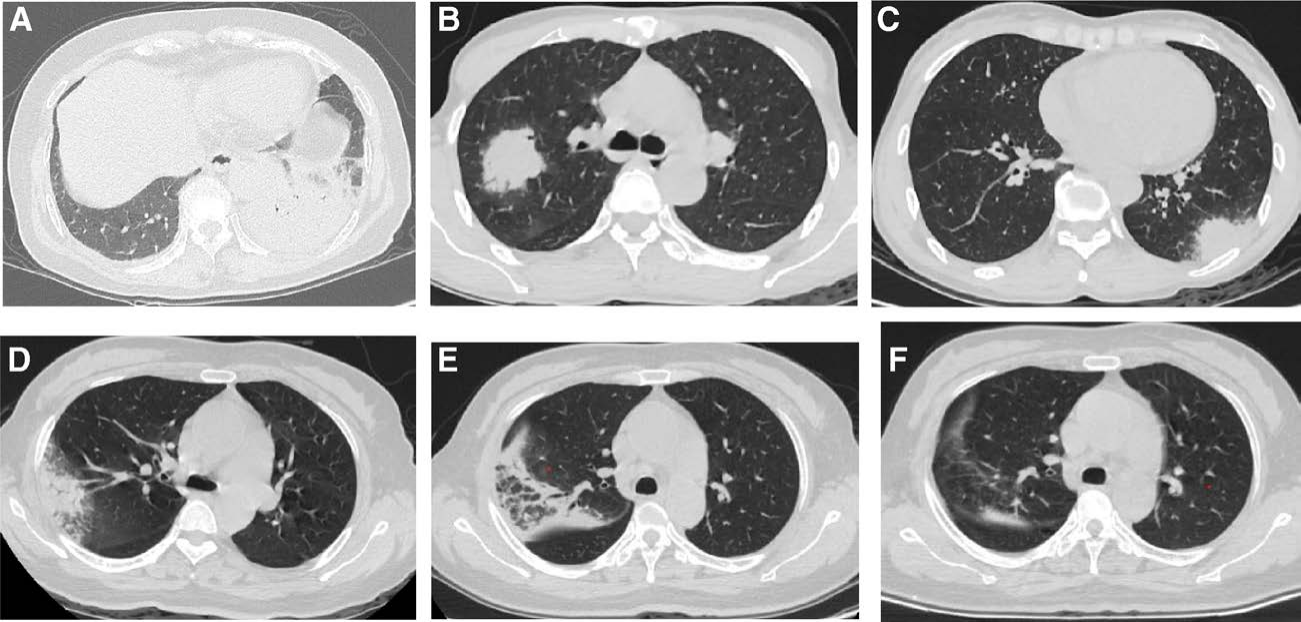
Chest computed tomography (CT) findings of non-severe *Chlamydia psittaci* pneumonia. (A) A 67-year-old female presenting a fever for 3 days, large air-space consolidation in the left lower lobe. (B) A 51-year-old male presenting a fever for 3 days, focal consolidation in the right upper lobe. (C) A 48-year-old female presenting a fever for 2 days, focal consolidation in the left lower lobe subpleural area. (D–F) A 51-year-old female with the initial CT scan performed 3 days after onset showed focal consolidation in the right upper lobe subpleural area (D). The lesions increased, and the scope expanded following treatment with levofloxacin for 7 days (E). Thereafter, the lesion evidently shrank and became less dense following treatment with moxifloxacin for 7 days (F).
